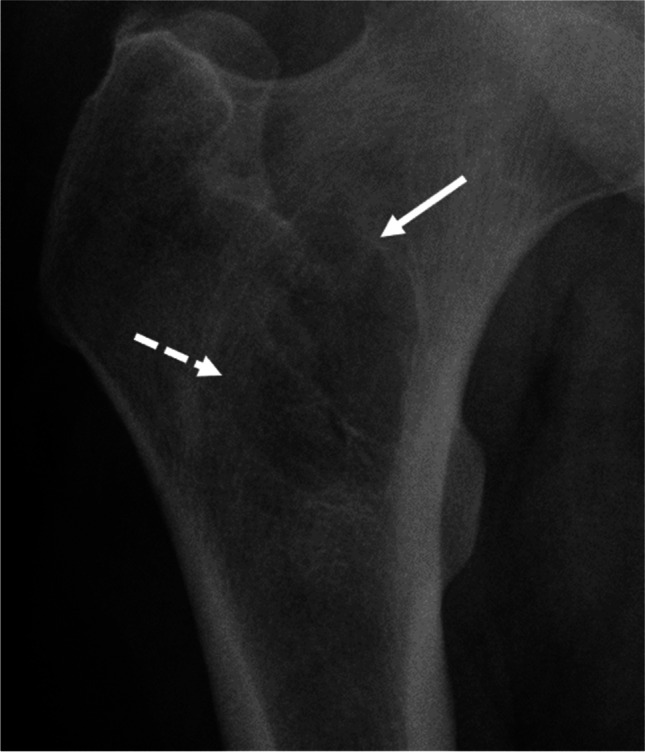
Grade IB. A 43-year-old man. A well-defined tumor is observed in the proximal femur. No penetration of cortex is evident. A faint sclerotic margin is observed in parts of the lesion (solid arrow); other parts of the lesion do not show a sclerotic margin (dashed arrow). There is no expanded shell. Because the sclerotic margin is not visible around the entire lesion, Lodwick growth grade IB is assigned. MRI was performed for further evaluation; the lesion proved to be a lipoma
